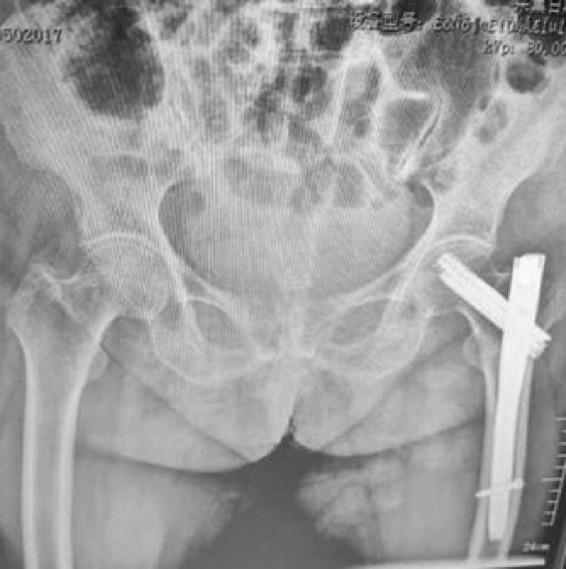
Postoperative anteroposterior radiograph demonstrating successful InterTAN fixation with anatomical fracture reduction and optimal implant positioning. The dual-screw configuration is properly aligned within the femoral head and neck.
